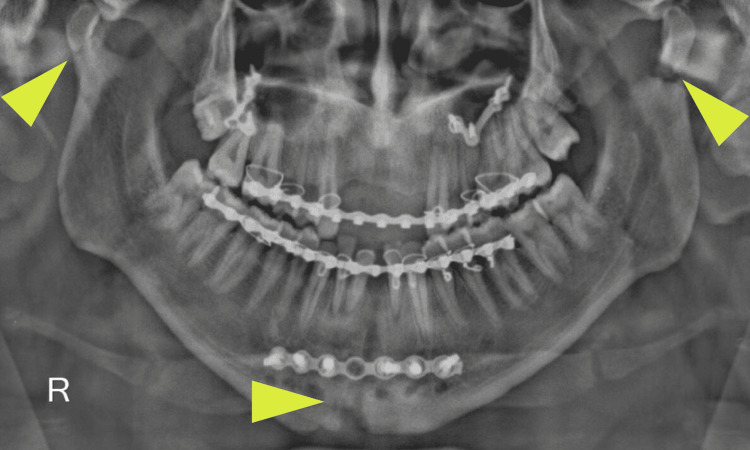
A postoperative orthopantomogram revealing bilateral mandibular subcondylar osteotomy (arrow) and vertical symphyseal osteotomy (arrows) for correction of post-traumatic deformity
